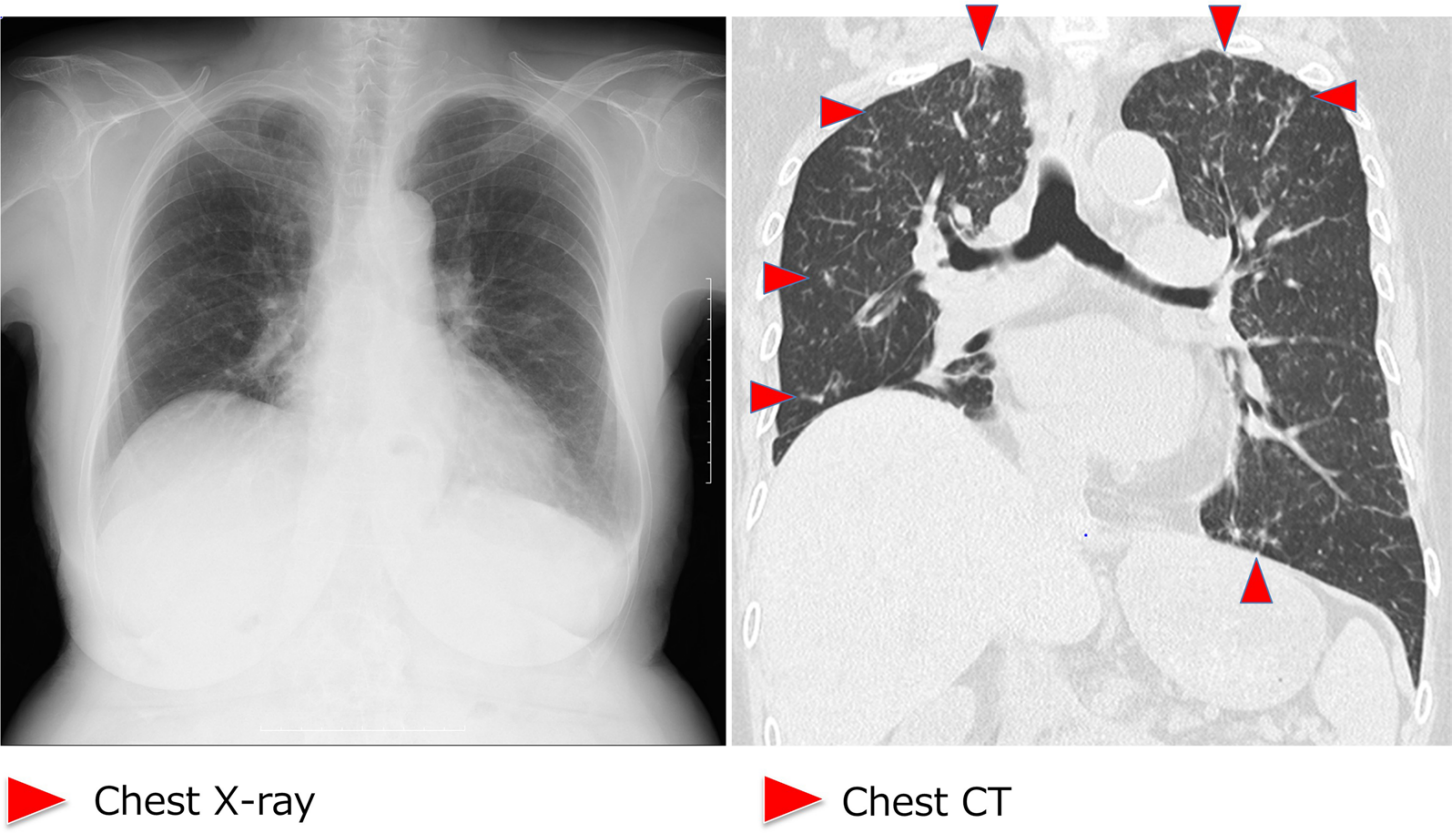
Chest X-ray and chest CT imaging of the non-tuberculous *Mycobacterium*-infected lungs. Images show multiple granular infiltration in the pleural side of all lung fields. Red arrow-heads indicate infiltration
